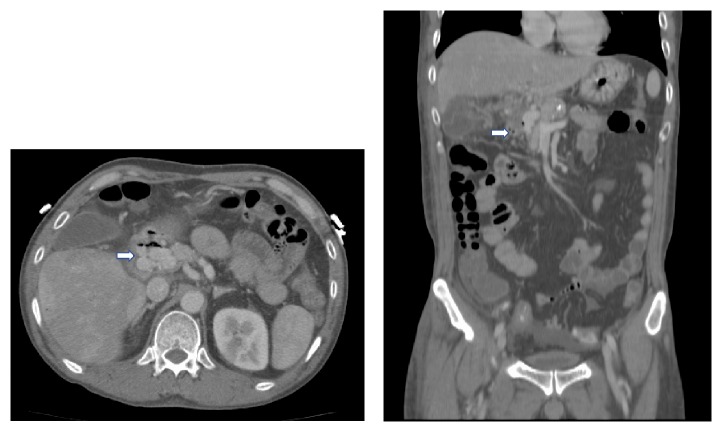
CT scan (coronal and axial views) showing multiple venous collaterals at the porta hepatis.
